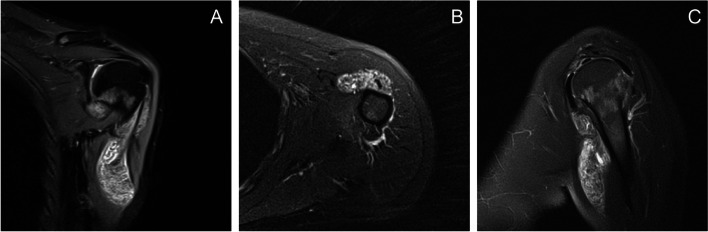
Preoperational MRI shows the massive lesion around the tendon of LHB and infiltration out of shoulder joint. A. The lesion on coronal section; B. The lesion on transverse section; C. The lesion on sagittal section. MRI, magnetic resonance imaging; LHB, long head of biceps
